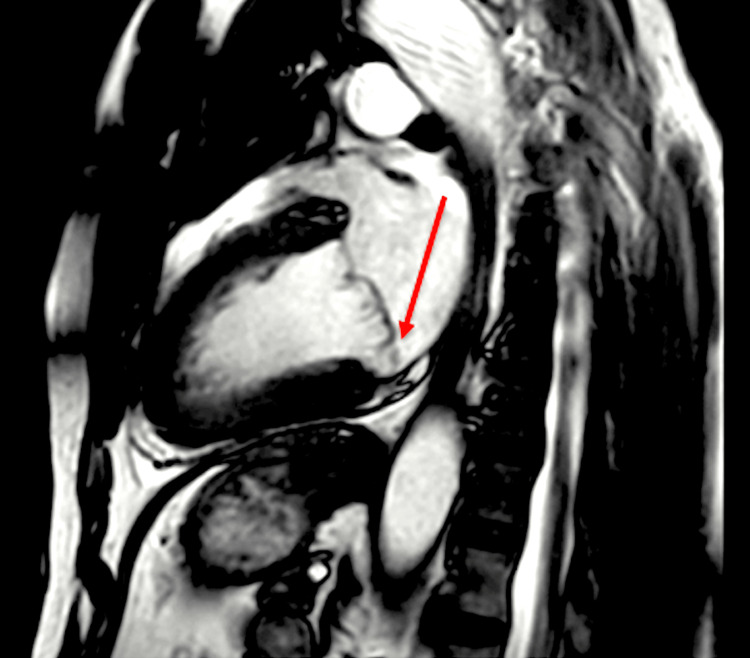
Cardiac MRI with an arrow indicating mild thickening and mild prolapse of the anterior mitral valve leaflet (predominantly A2 scallop) MRI: magnetic resonance imaging
